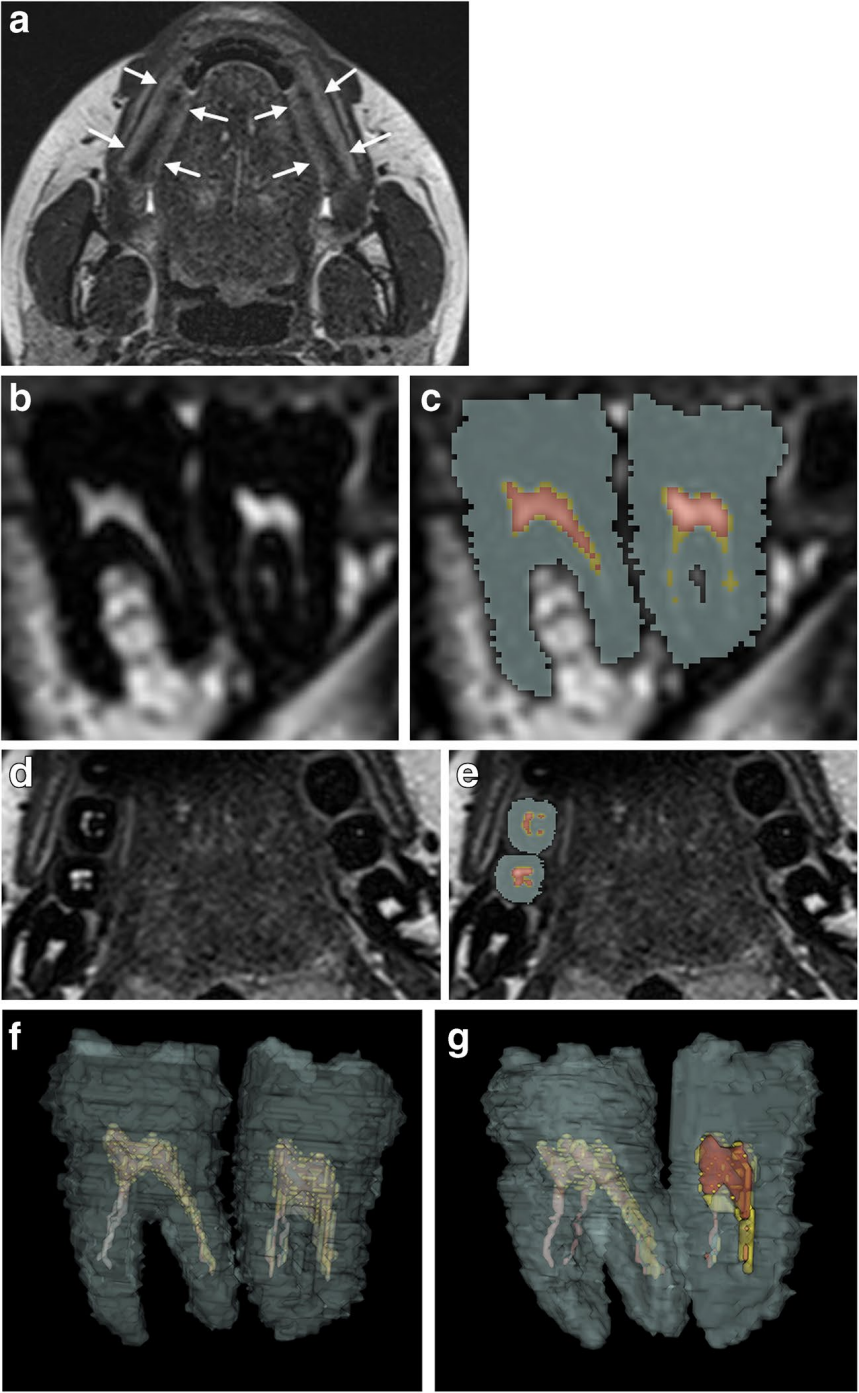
Axial MRI shows correct bilateral placement of dental cotton rolls soaked in water (arrows) between the molars (a). The cotton rolls delineated the upper and lower molars and stabilized the bite. Axial MRI through the upper jaw shows unsegmented (b) and segmented (c) tooth 26 and 27. Grey for hard tooth tissue, yellow for low signal soft tissue and red for high signal soft tissue. Lower and upper thresholds were set at 0 and 63 for hard tooth tissue, 64 and 100 for low signal soft tissue and ≥101 for high signal soft tissue. Sagittal MRI through the upper jaw shows unsegmented (d) and segmented (e) tooth 26 and 27. Grey for hard tooth tissue, yellow for low signal soft tissue and red for high signal soft tissue.3D of the entire tooth 46 and 47 (f) and 3D rendering of the segmentation (g), with a wedge of approximately one half removed to visualize the high signal soft tissue. Grey for hard tooth tissue, yellow for low signal soft tissue and red for high signal soft tissue
